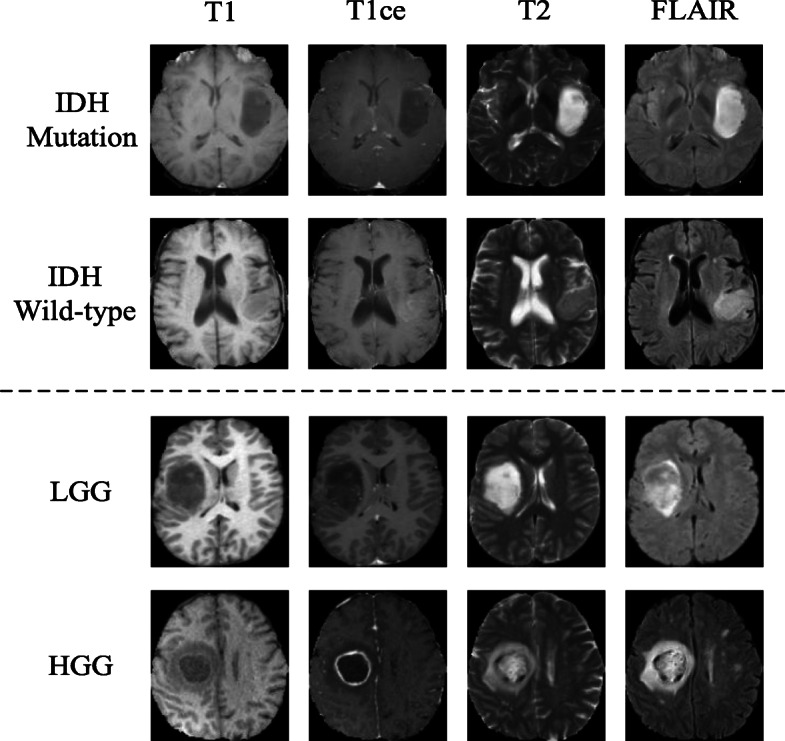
Examples of 2D MRI slices from IDH mutation/wild-type gliomas and LGG/HGG in four modalities
